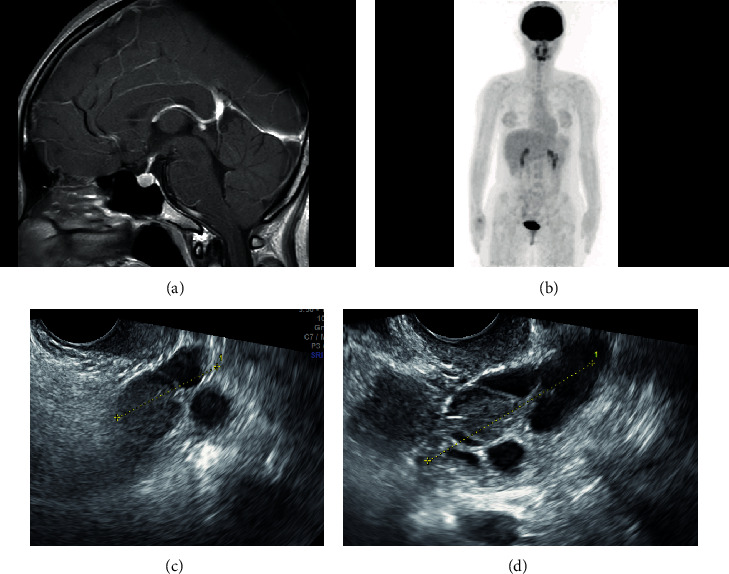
(a) Pituitary magnetic resonance imaging (gadolinium-enhanced T1-weighted sagittal image). No tumor is observed in the pituitary gland. (b) Result of positron emission tomography. No ectopic tumor is observed. (c, d) The left ovary after estrogen/gestagen therapy was discontinued. (c) Cycle date 3: 3.0 cm. (d) Cycle date 9: 4.8 cm.
